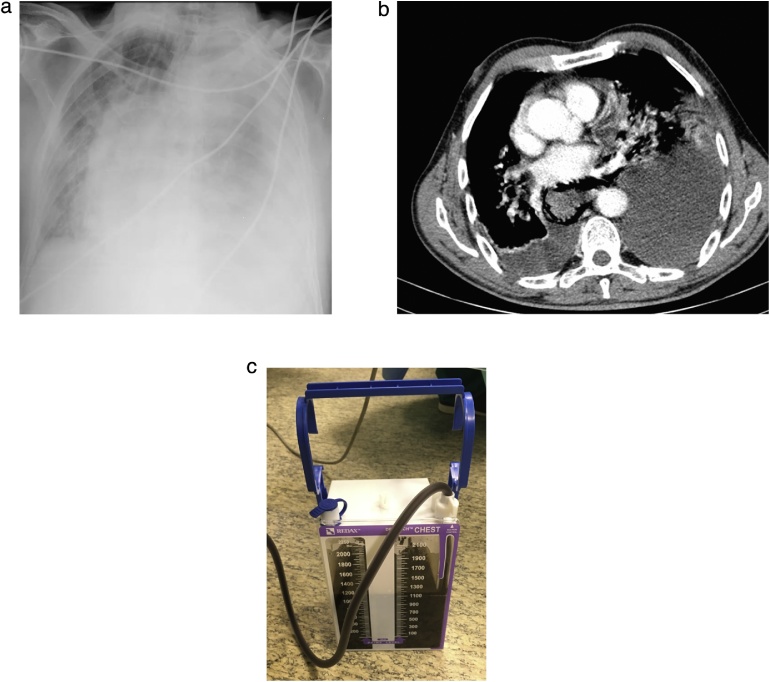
Diagnosis progress. A. Chest x-ray – left pleural effusion. B. CT scan – pneumomediastinum, left collapse lung and heterogeneous pleural effusion. C. Chest tube drainage – food residue.
